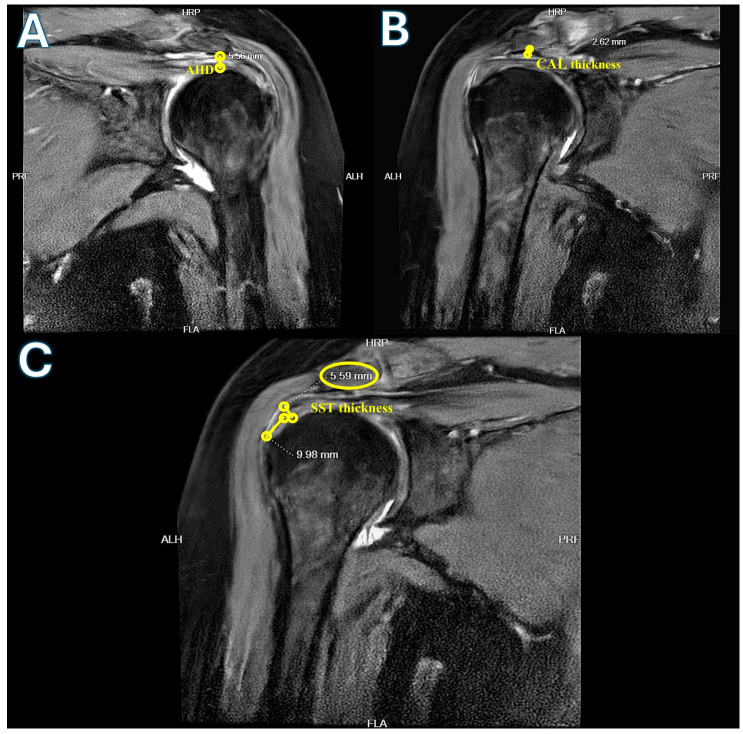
Distance and soft tissue measurements on shoulder MRI. (A) Acromiohumeral distance (AHD), defined as the shortest distance between the inferior surface of the acromion and the humeral head, measured on a coronal oblique T1-weighted MRI image. (B) Coracoacromial ligament (CAL) thickness measured at the thickest portion of the ligament on a sagittal oblique T2-weighted MRI image. (C) Supraspinatus tendon (SST) thickness measured approximately 10 mm lateral to the biceps tendon on a coronal oblique T2-weighted fat-suppressed MRI image. HLP, ALH, PRF, and FLA represent orientation markers automatically generated by the MRI system.
